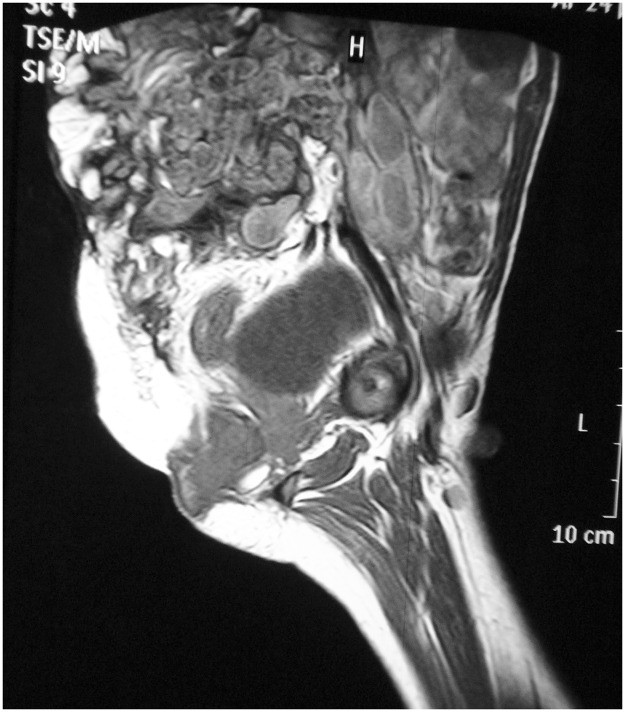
MRI showing an aggressive infiltrating lesion invading the pelvis and lumber region that extends from the posterior abdominal wall, with a sizable intrapelvic and intraabdominal extension infiltrating the lumber and sacral vertebrae and an intraspinal epidural extension displacing the abdominal organ to the left; in addition, there were many “dot-in sign” of mycetoma.
